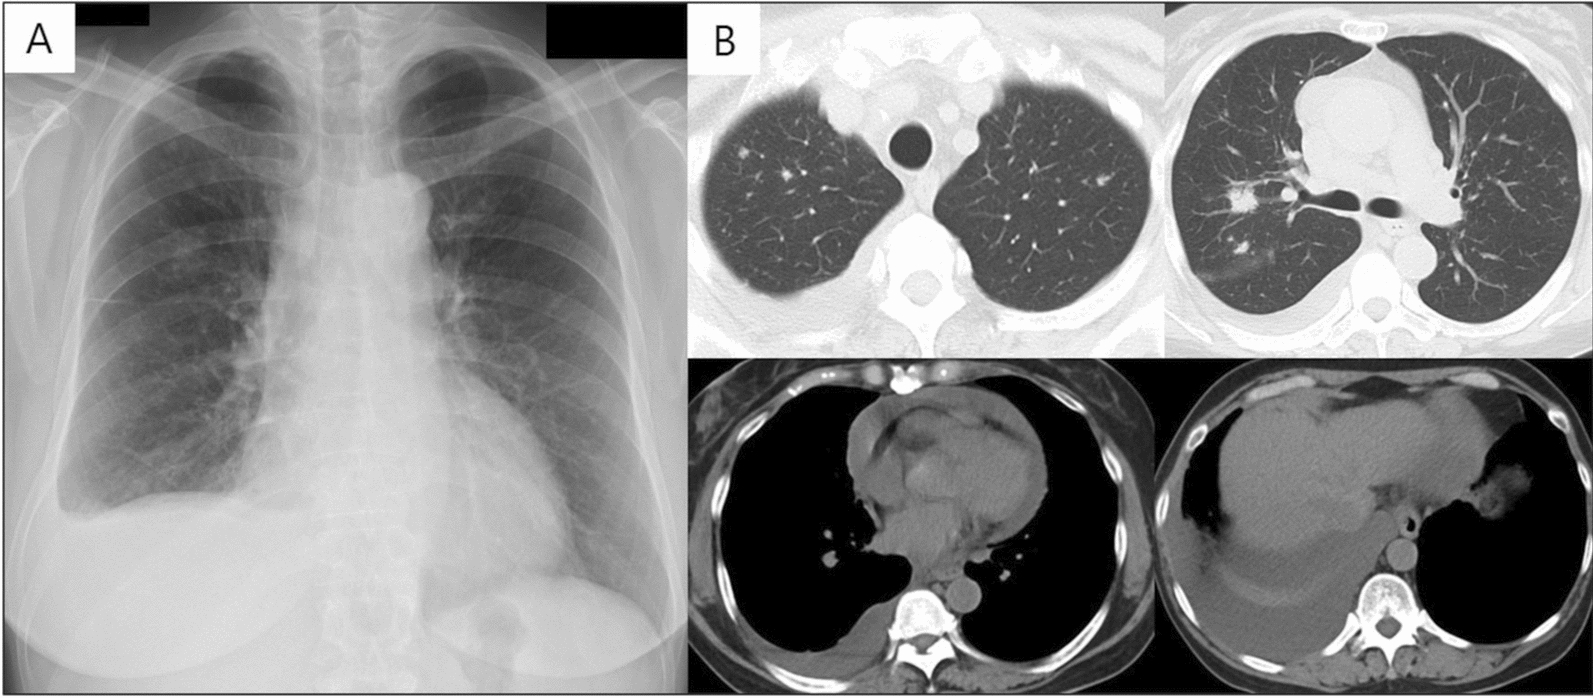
A Chest radiograph showed infiltrative shadows and small nodular shadows in the upper right middle lung field. The right costophrenic (CP) angle was dull. B A computed tomography scan showed multiple nodules in both the upper lobes and right pleural effusion and pericardial effusion
